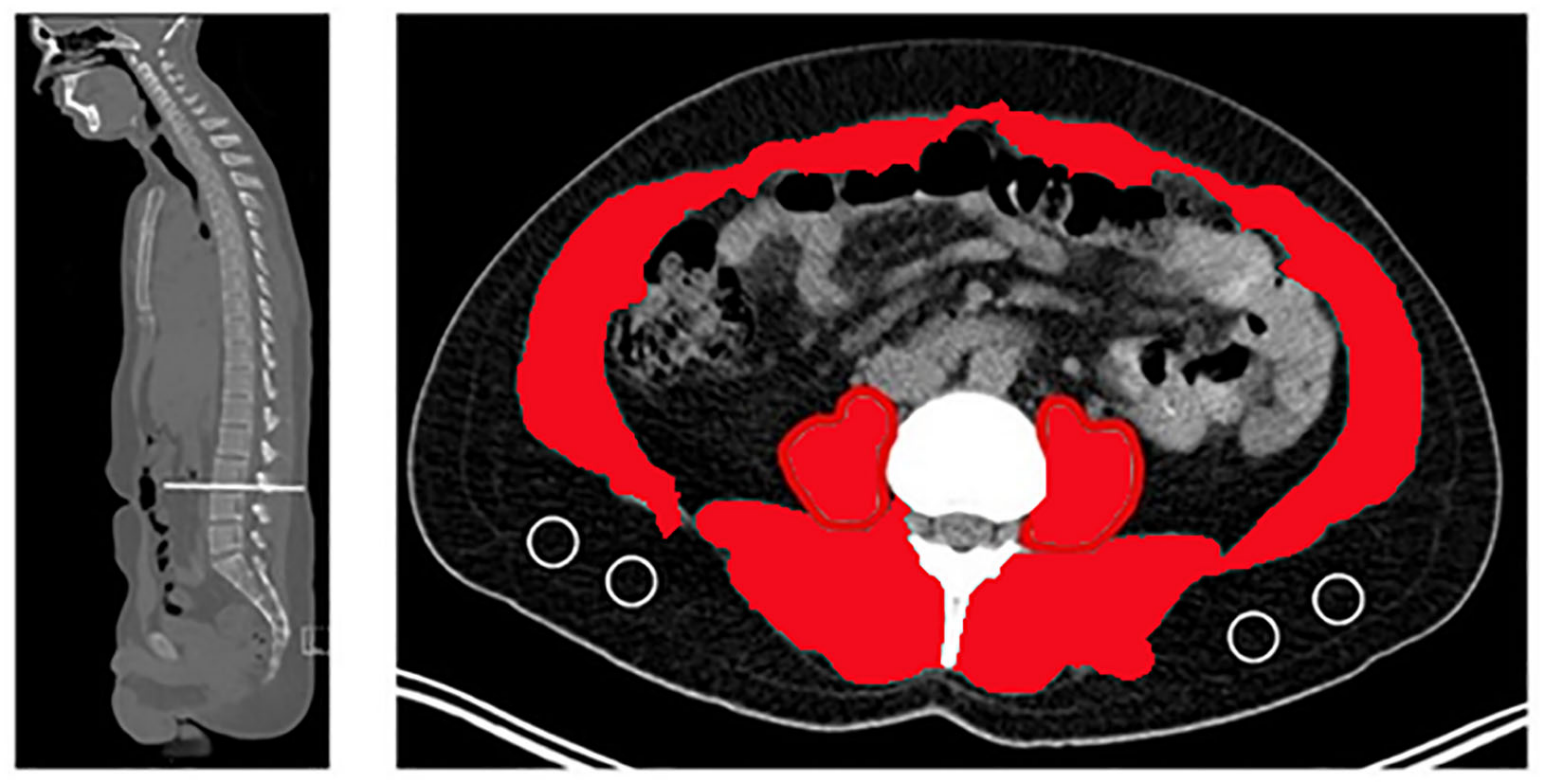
Cross-sectional computed tomographic images at the L3 level.
